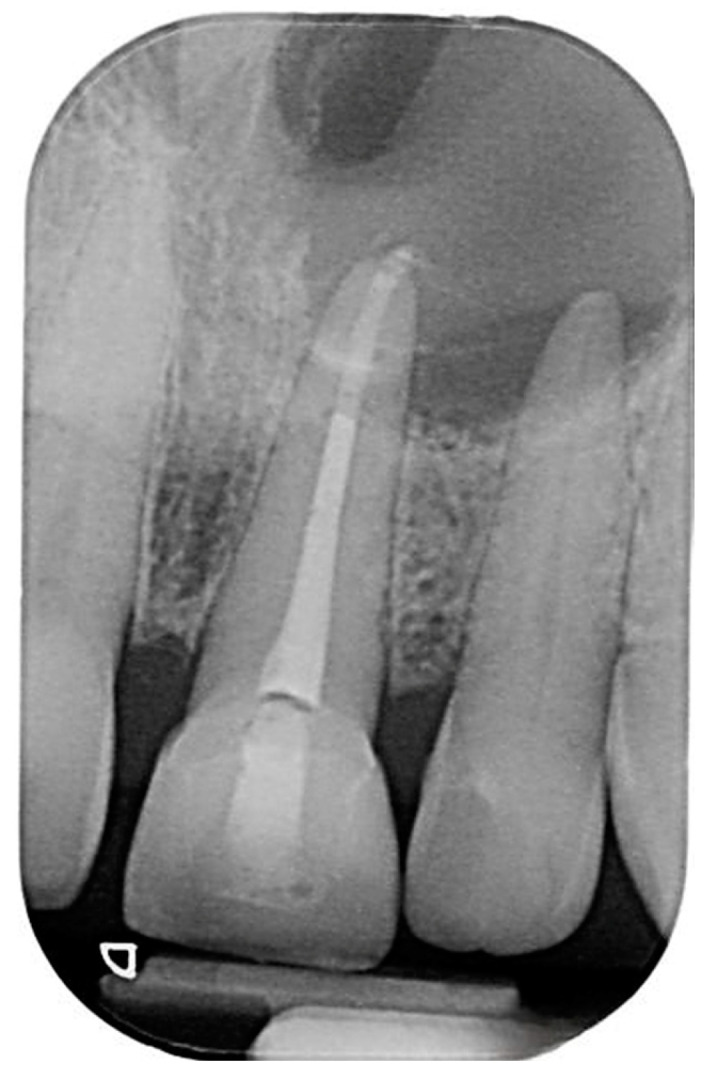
Postoperative intraoral periapical radiograph the upper left central and lateral incisors.
